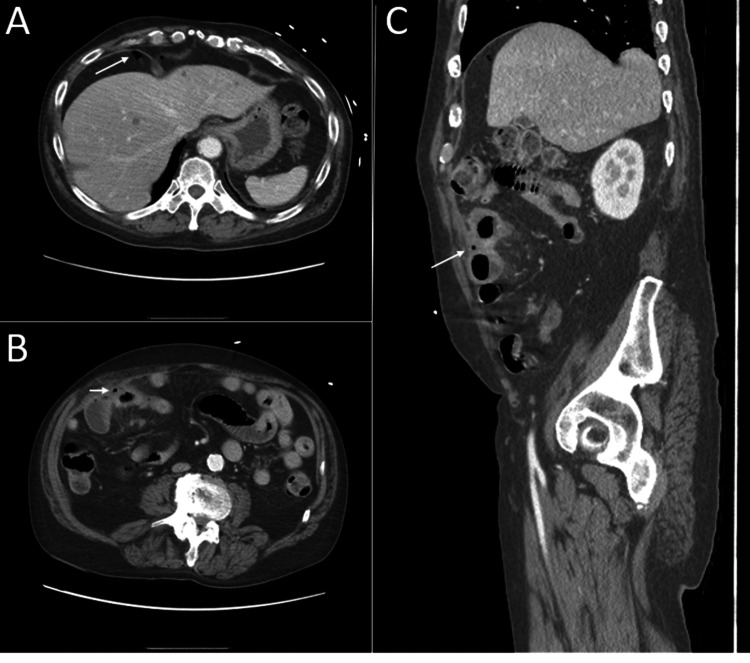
CT of the abdomen and pelvis with IV contrast (A) Axial view showing free air pocket under the right diaphragm. (B) Axial view showing small extraluminal air pocket and fluid collection between small bowel loops and wall of the right lower quadrant. (C) Sagittal view showing pocket of free air and fluid collection.
